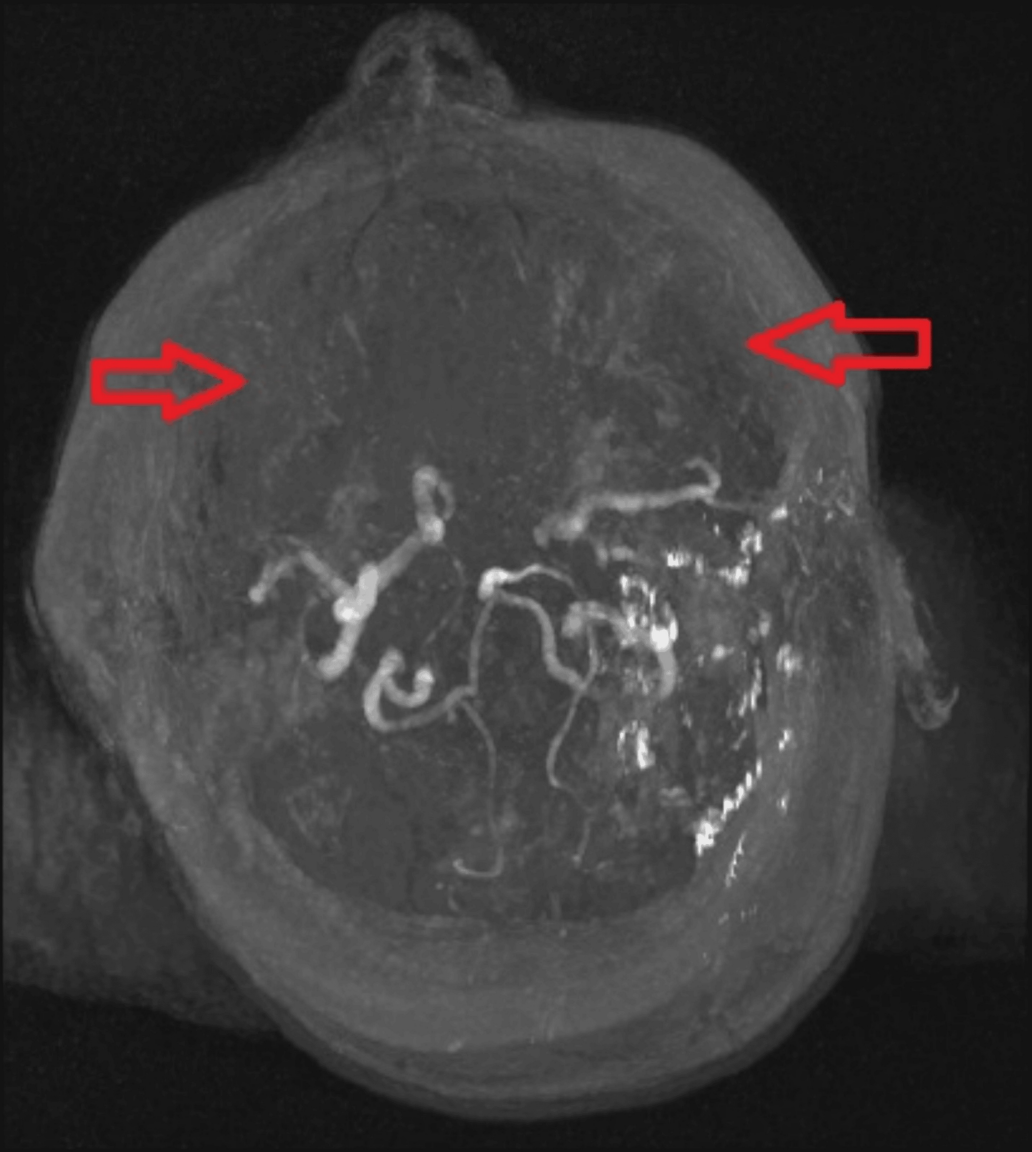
MRI imaging showing "puff of smoke" characteristic of Moyamoya disease
